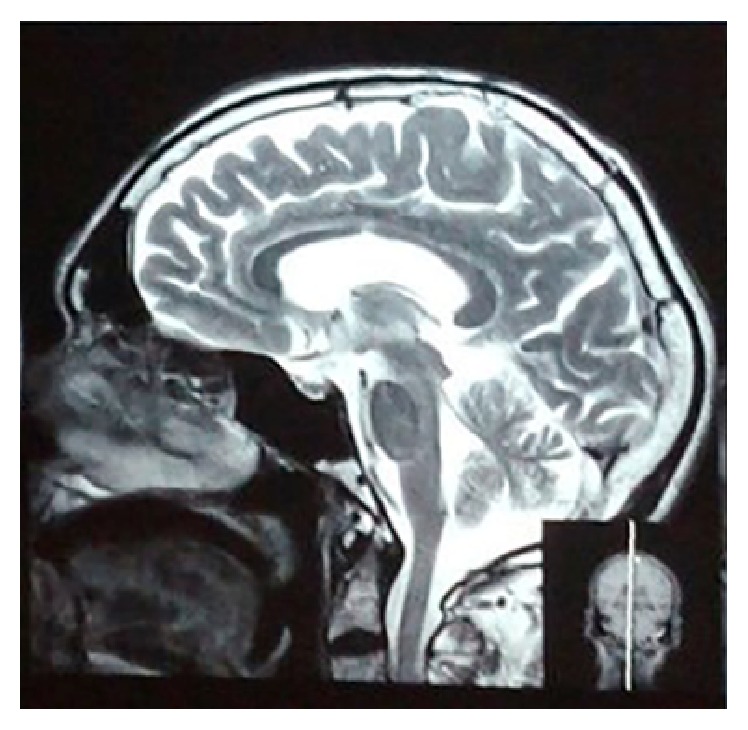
Brain MRI showing cerebral and cerebellar atrophy.
